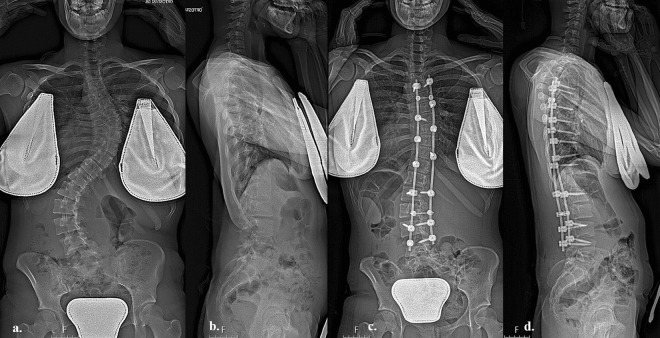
(a) Anteroposterior pre-operative long-cassette X-Ray of a 6 C-Adolescent Idiopathic Scoliosis in a seventeen years patients with Risser 5; (b) lateral pre-operative long-cassette X-ray showing hypokyphosis < 20°, (c) post-operative anteroposterior long-cassette X-ray showing satisfactory correction with all screw construct, (d) lateral post-operative long-cassette X-ray showing restoration of better kyphosis.
